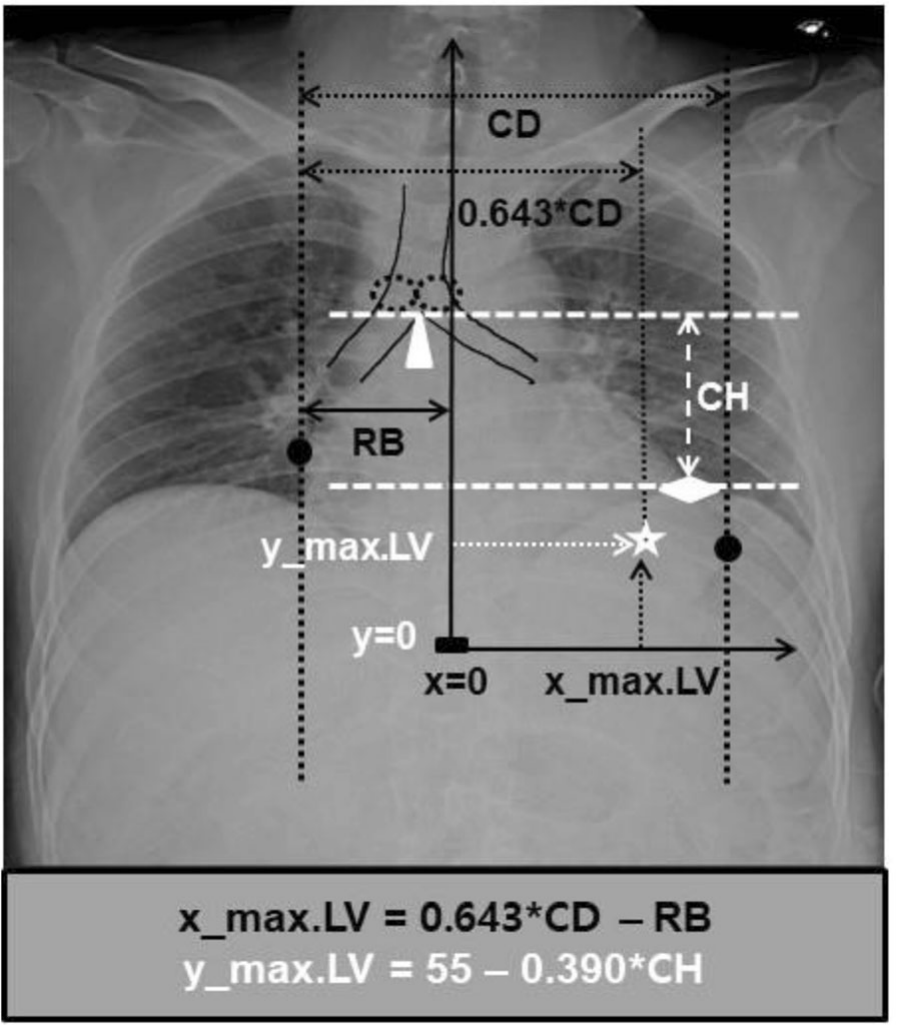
Estimation of the theoretical optimum chest compression point from anteroposterior chest radiography (modified from a figure of reference 1)
